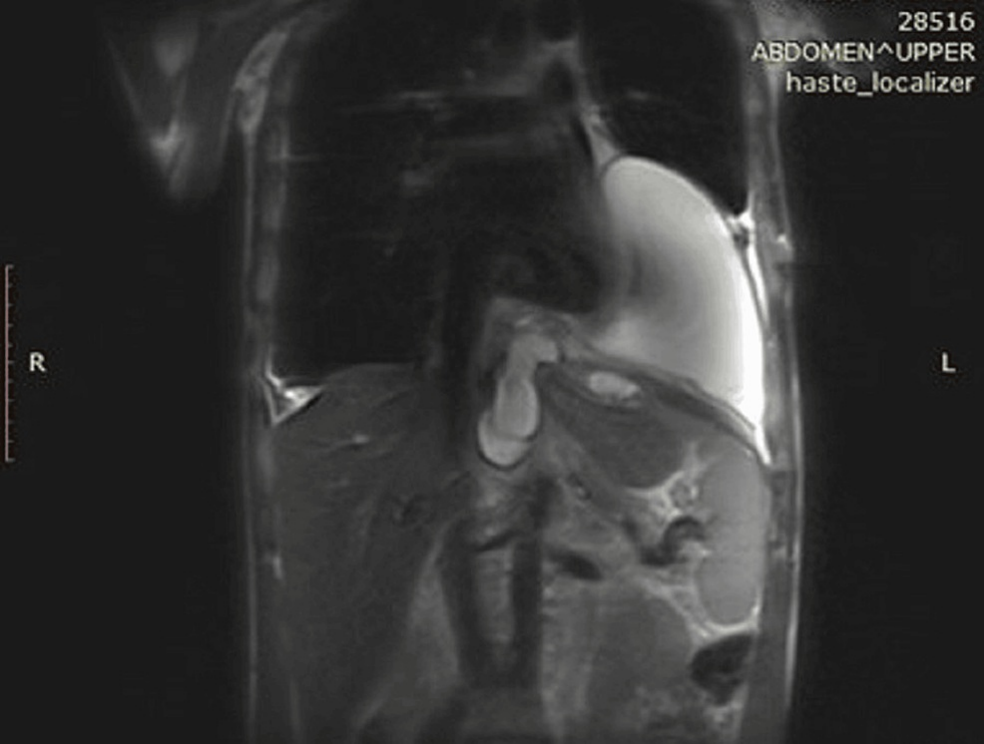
The pancreatic cysts, invading the mediastinum and left pleural cavity as seen on MRI
